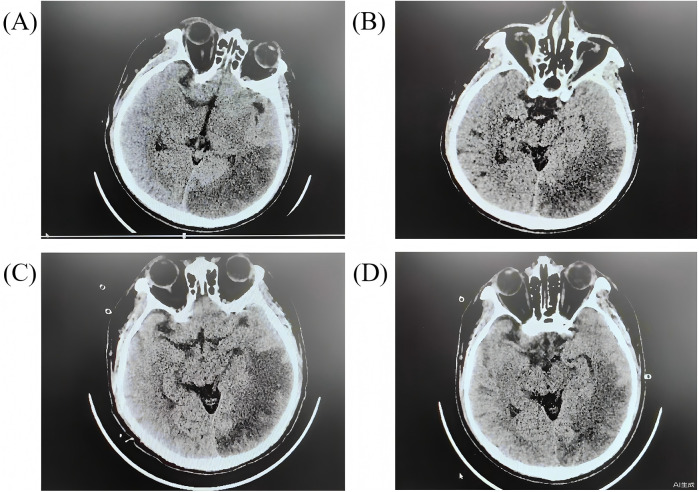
The head CT scans for the patients during hospitalization. (A) day 2 after arrest; (B) day 7 after arrest; (C) day 11 after arrest; (D) day 15 after arrest.
